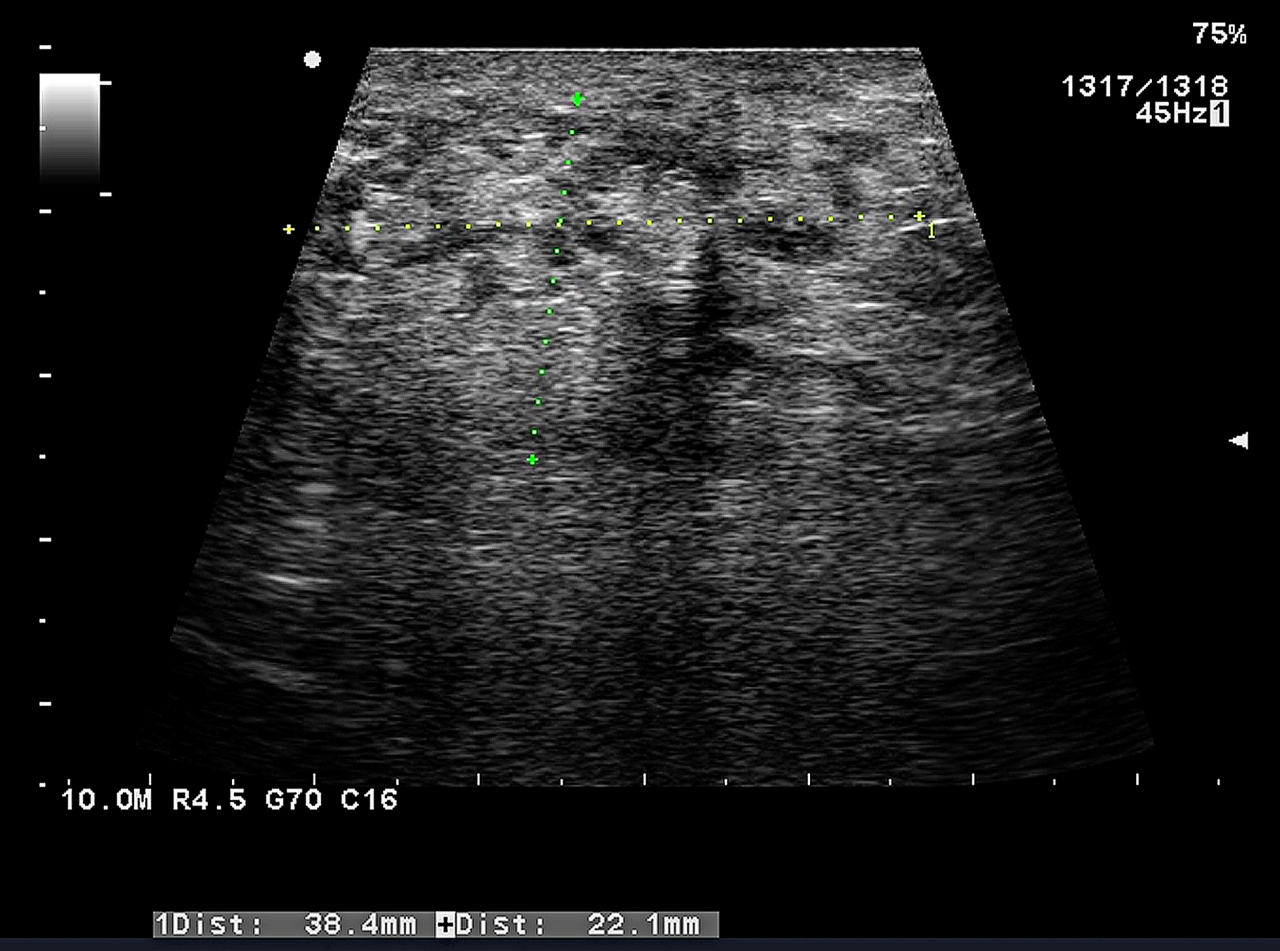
Ultrasound examination of the contents of the patient’s scrotum (in this case, the right testicle) performed prior to the final indication for surgery. According to the final evaluation, it was determined that it is a malignant tumour developing from testicular tissue that also extends into the epididymis, causing induration in this location. As previously noted in the text, the dilation of the veins of the pampiniformis plexus of the first degree on the left was a secondary finding. No additional pathologies were discovered. This was the decisive examination on the basis of which the patient was indicated for orchiectomy.
